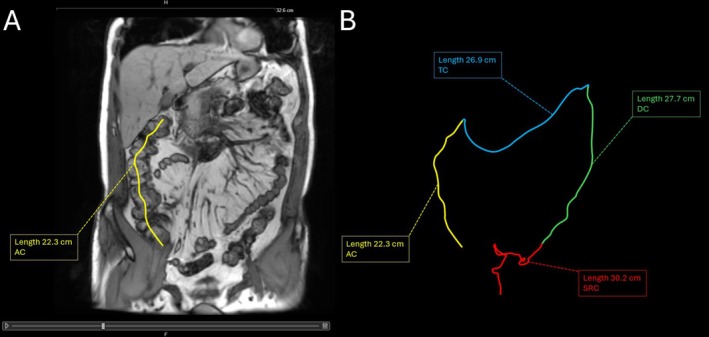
(A) Example of length measurements for the ascending colon (AC) segment of a participant. The figure displays one MRI coronal image plane of the multi‐slice stack with the yellow AC length measuring line projected on top. (B) The measuring lines for the entire colon length, comprising the four individual measuring lines for the AC (in yellow), transverse colon (TC, in blue), descending colon (DC, in green), and sigmoid‐rectum colon (SRC, in red). The measuring lines are manually drawn in three dimensions on the coronal multi‐slice MRI stack and displayed projected on the two‐dimensional plane.
